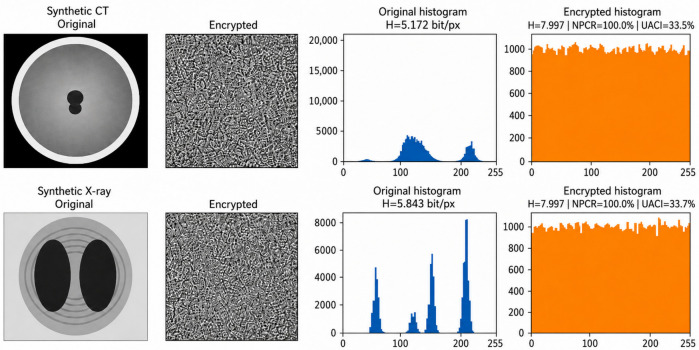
S-box encryption of synthetic CT and X-ray images: noise-like ciphers, flattened encrypted histograms, NPCR = 100%, UACI ~33.5%.
